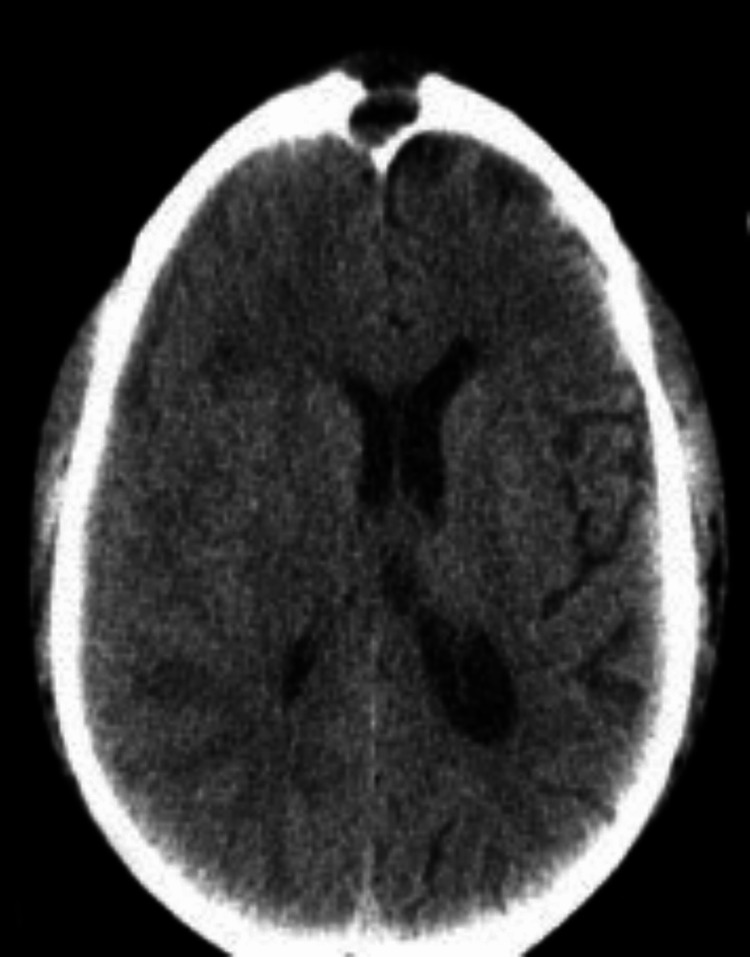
Axial CT without contrast, demonstrating erosion of walls of the frontal sinus.
